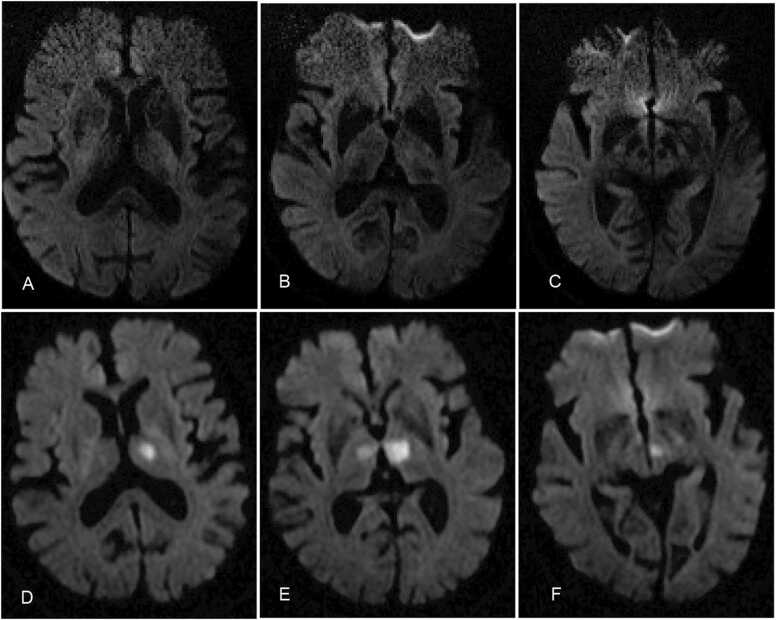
Diffusion-weighted MRI scans of Case 2 patient with AOP infraction. Diffusion weighted images A–C does not display abnormal diffusion-limited signals on initial presentation; D–F, images after review of increased disturbance of consciousness, showed hyperintense signals in the bilateral paramedian thalami and paramedian.
